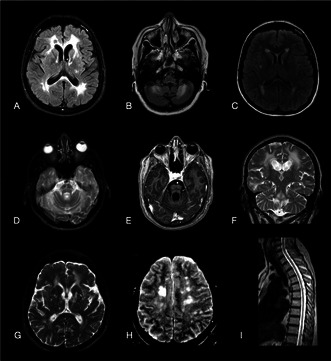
FIGURE 1 MRI scans from 9 cases analyzed in this study.
